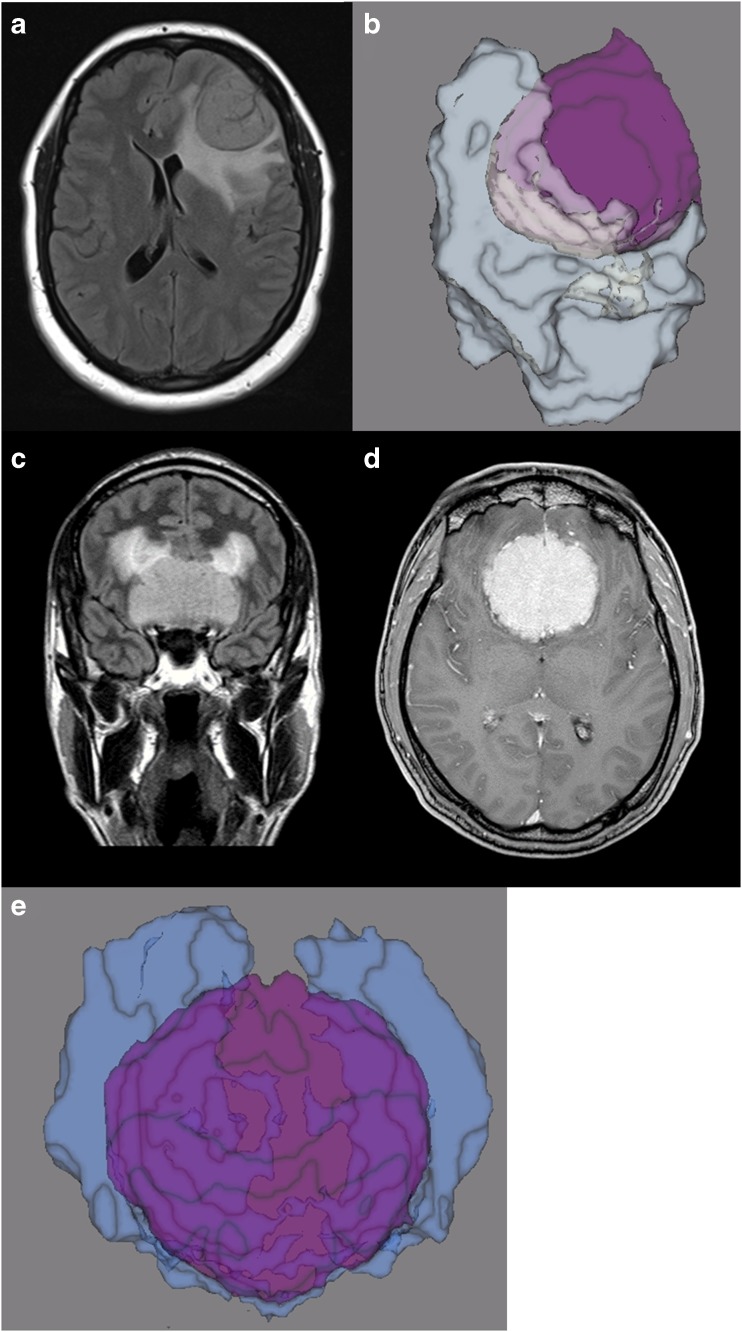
Three-dimensional rendering of two segmented tumour volumes. a, b Patient from Fig. 3, a 53-year-old woman with a meningioma grade II in the left frontal lobe with wide dural attachment. c, d, e A 33-year-old man with a grade I meningioma of the falx with great tumour volume in both frontal lobes and surrounding oedema in the adjacent white matter. Dice coefficients were 0.89 for TTV and 0.92 for T1CE TV. a and c FLAIR images; d T1CE MR image. b and e Three-dimensional rendering of the two meningiomas; contrast-enhancing tumour parts are displayed in *purple* and surrounding oedema in *white and blue*
